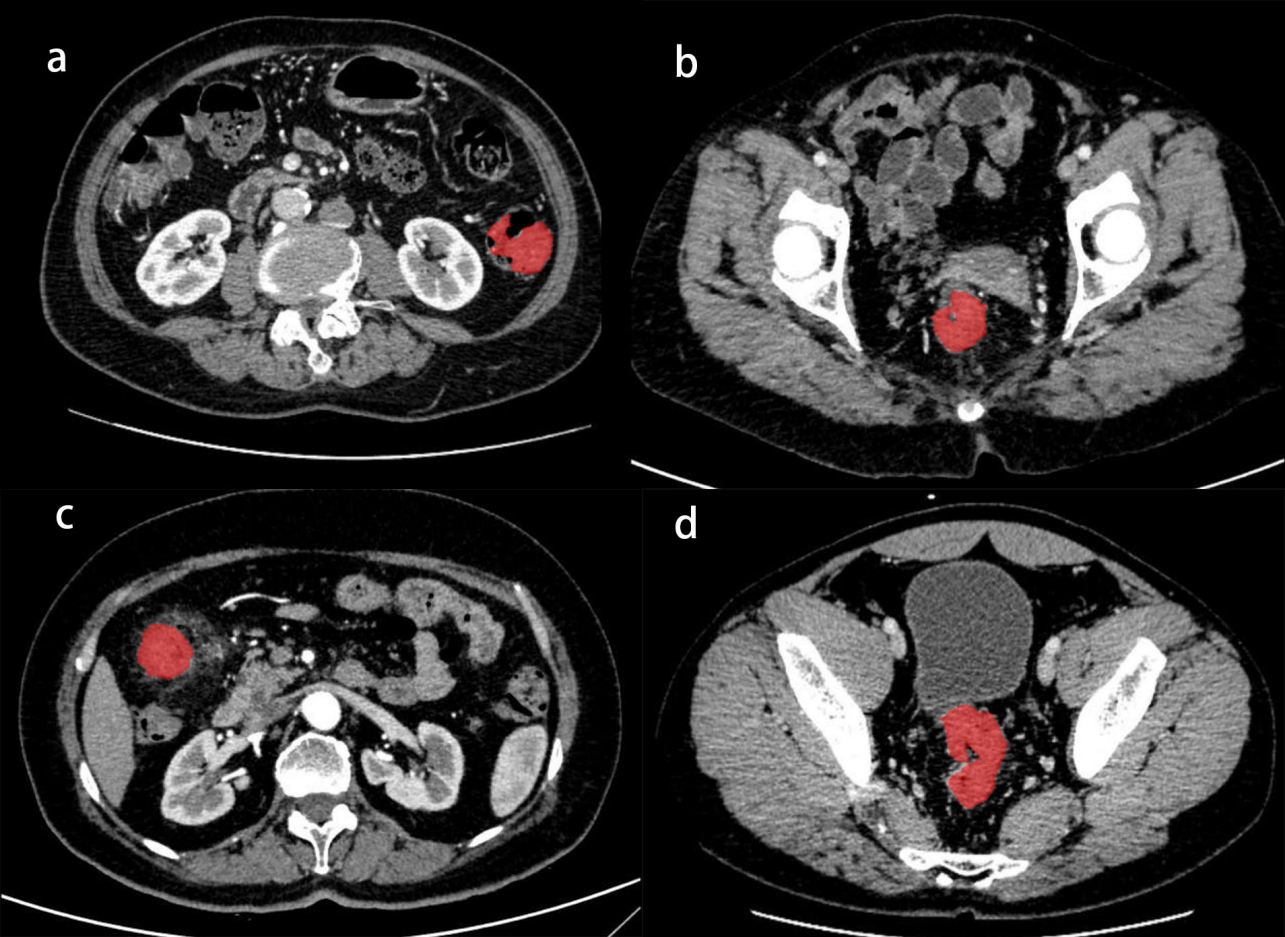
The selection of ROI of CT images using MaZda software. (A) ROI of the mass in the descending colon; (B) ROI of the mass in the rectum; (C) ROI of the mass in the ascending colon; (D) ROI of the mass in the sigmoid colon.
